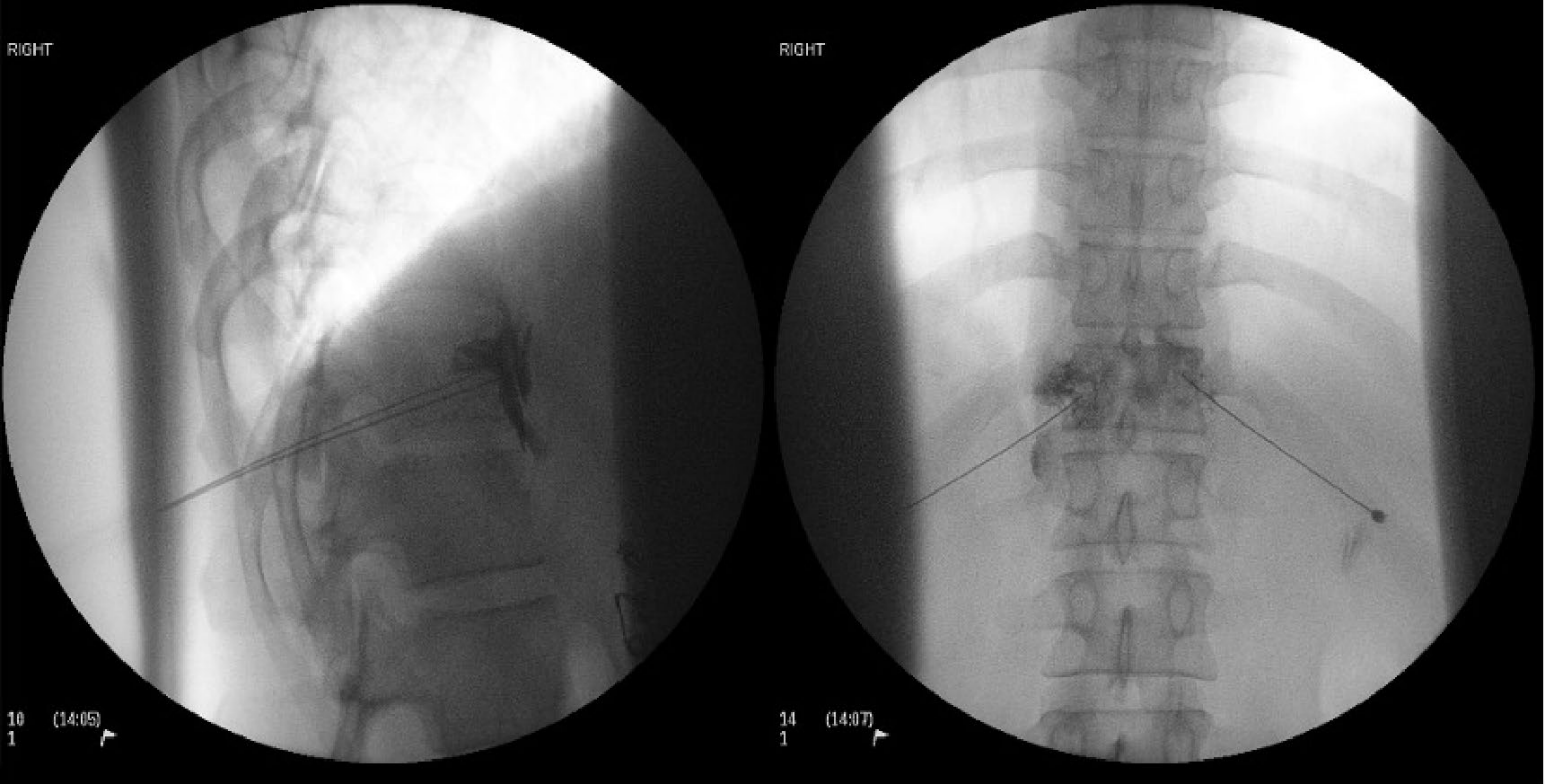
Images from splanchnic nerve block with fluoroscopy/Xray guidance
